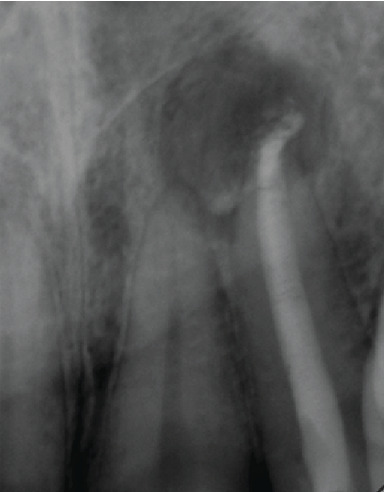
Periapical radiograph showing that tooth 22 had a well-condensed root canal filling, where the gutta percha was overextended with a periapical lesion at the area.
